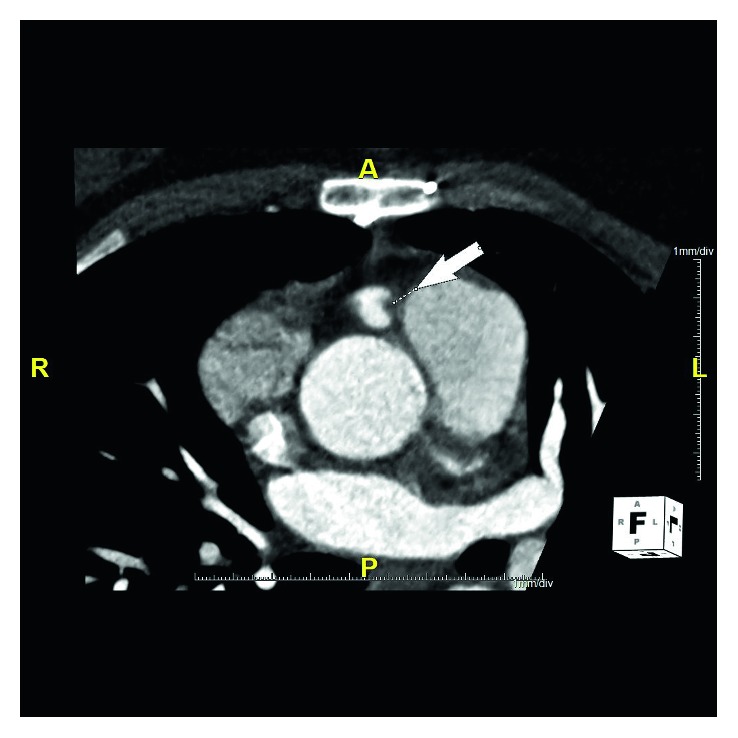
Cross-sectional view of the coronary CTA, after surgery, shows the filling defect in the implanted RCA consistent with partially occlusive thrombus.
